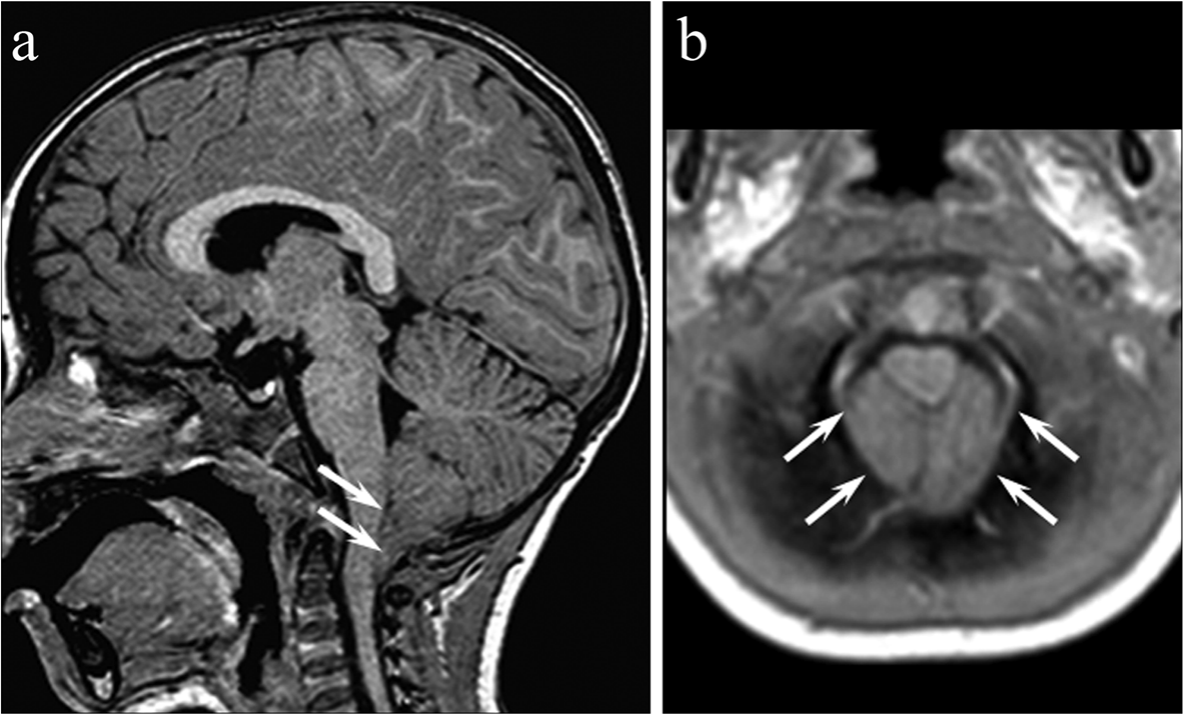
Sagittal contrast-enhanced T1-weighted (a) showing a caudal protrusion (12 mm) of the elongated triangular-shaped tonsils below the foramen magnum (white arrows). Axial T1-weighted image (b) confirms cerebellar tonsils filling the foramen magnum and effacing the cysterna magna (white arrows).
